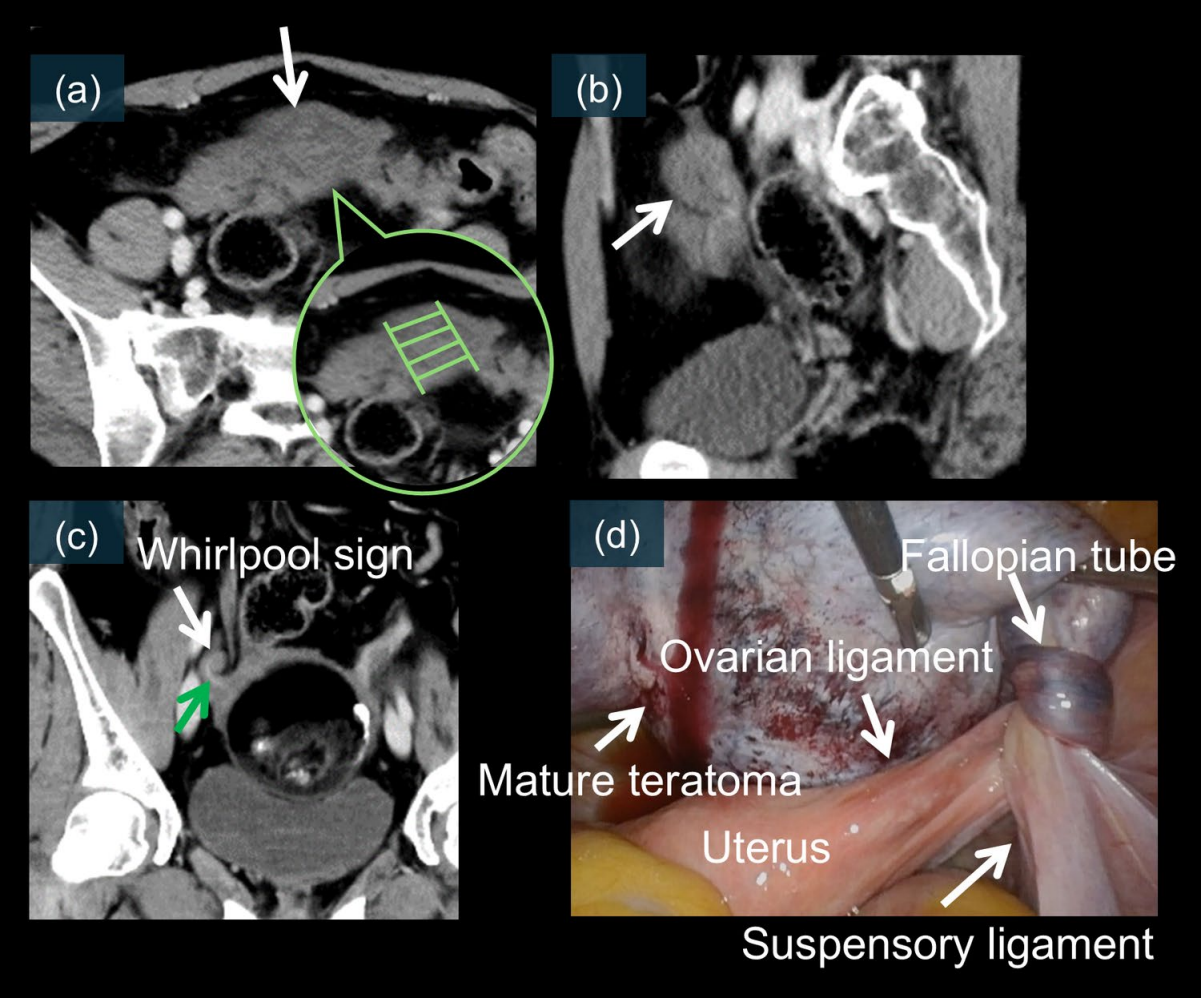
A 26-year-old female. a–c are axial, sagittal, and coronal contrast-enhanced CT images. The mature cystic teratoma is located in the midline of the pelvis. Although a clear whirlpool sign is not evident on the axial images, a step-ladder pattern of ovarian vessels cranial to the mature cystic teratoma is observed (a, white arrow). This structure is particularly well-defined on sagittal (b, white arrow) and coronal (c, white arrow) images. A whirlpool sign is visible, particularly on the coronal image, accompanied by an elongated enhancement suspected to be the ovarian artery within it (twisted vascular pedicle) (c, green arrow). d is a laparoscopic image, which reveals a 540° torsion of the right mature cystic teratoma
